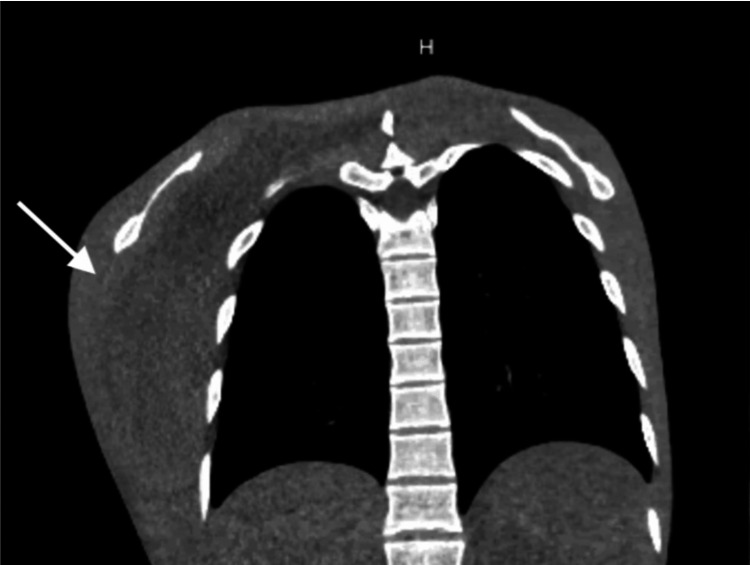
CT scan of the chest (coronal view) Right-sided chest wall hematoma (white arrow)
